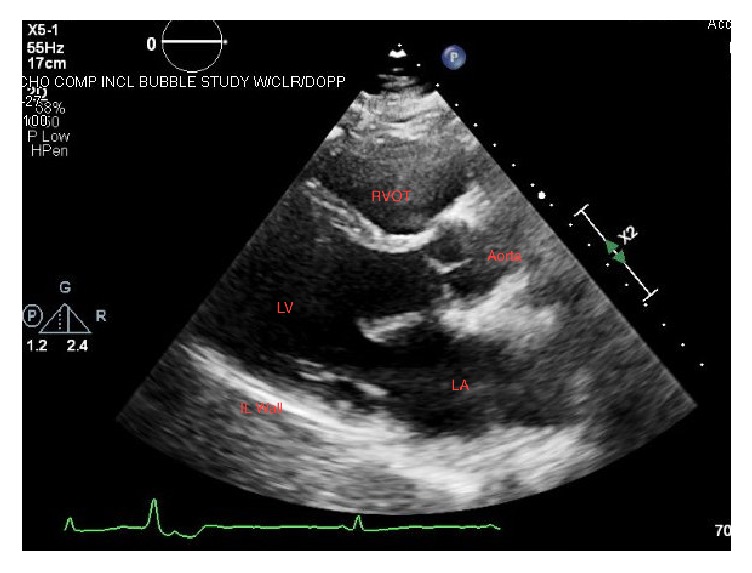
Transesophageal echocardiogram, parasternal long axis view. LA: left atrium. LV: left ventricle. RVOT: right ventricular outflow tract. IL wall: inferolateral left ventricular wall showing hypokinesis of ventricular myocardium.
